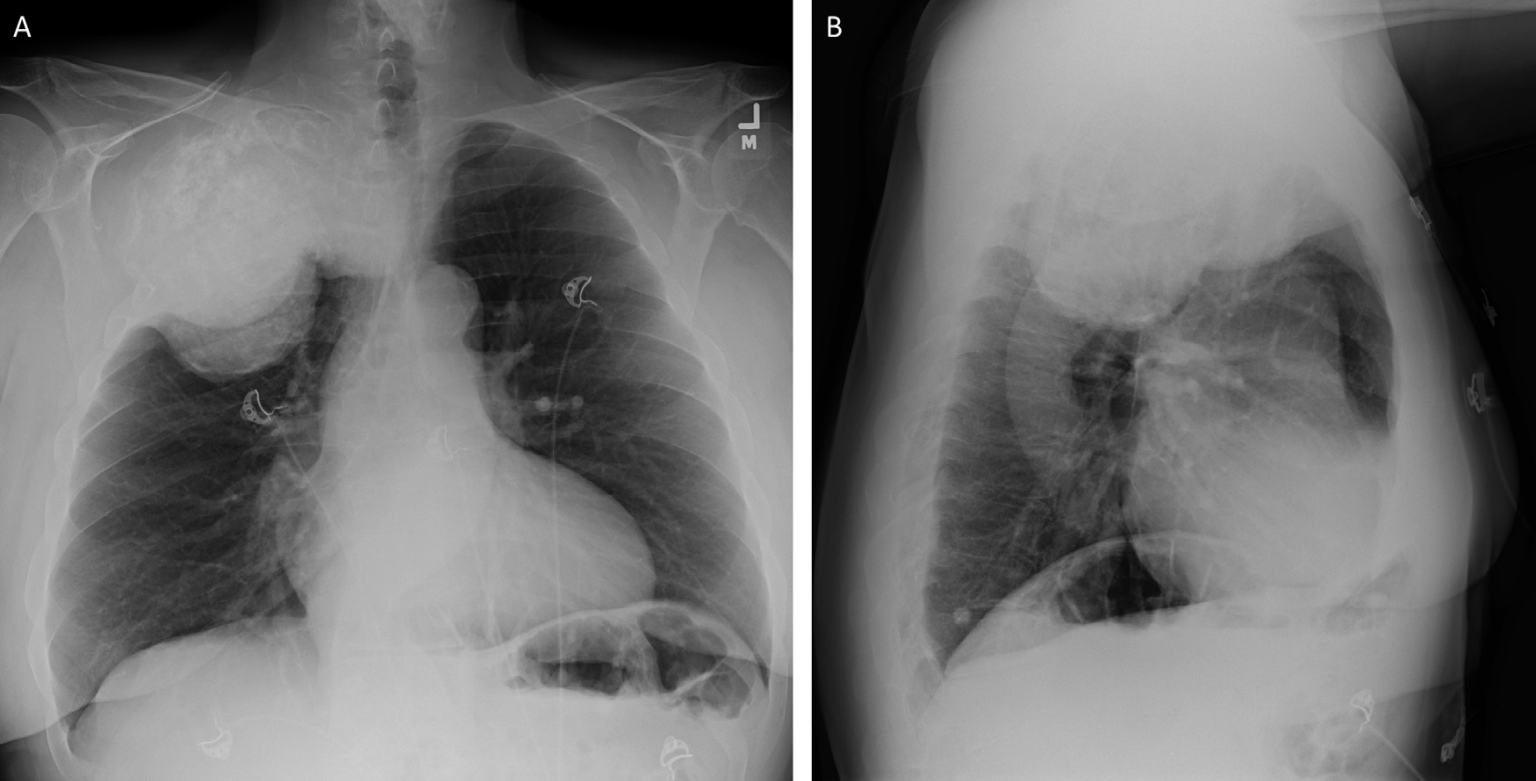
AP (A) and lateral projection (B) radiographs demonstrate a large right apical chest wall mass. This mass demonstrates foci of calcifications
